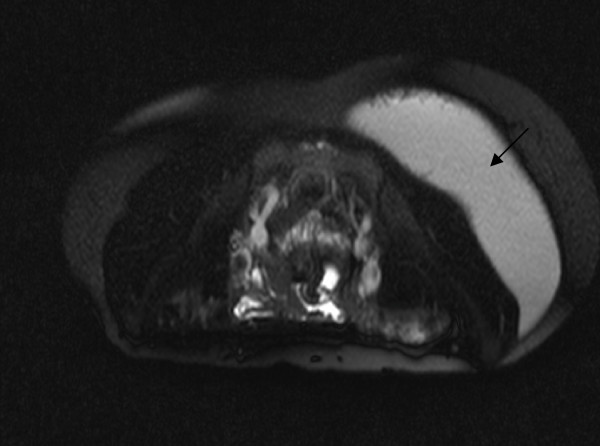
MRI in sagital plane showing the collection was superficial to the muscles of the back and the gluteal region but deep to the posterior layer of the thoraco-lumbar fascia.
